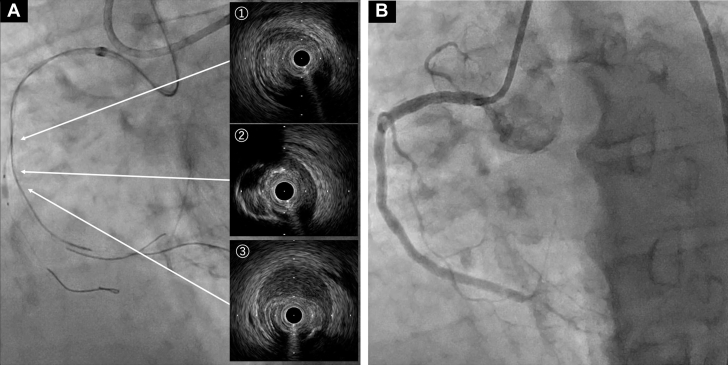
Prestent IVUS and Final Angiography (A) The Gladius wire was able to directly cross the CTO exit. AnteOwl IVUS revealed Gladius wire progression: (①) true lumen proximal to CTO, (②) subintimal space immediately after CTO entry, (③) re-entry into true lumen approximately 1 cm distal to CTO entry. (B) Final angiography image. CTO = chronic total occlusion; IVUS = intravascular ultrasound.
